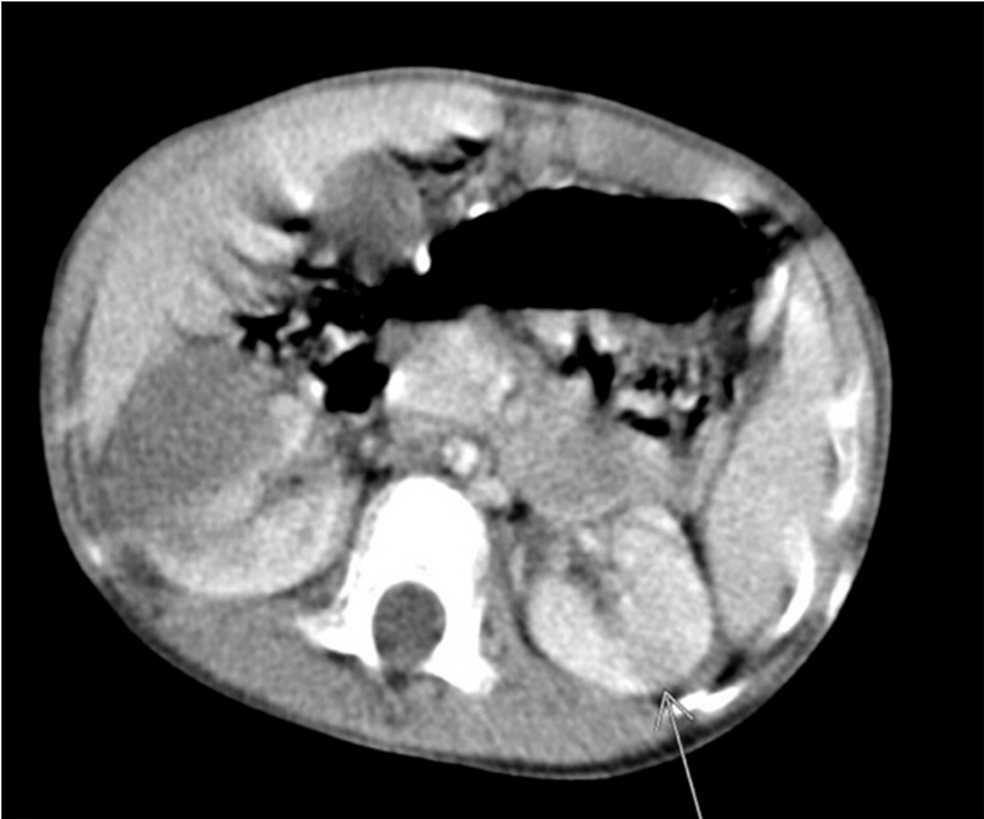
CT scan after six cycles of chemotherapy showed a marked reduction in the right renal tumor and minimal changes in the left tumor (arrow indicates mass in the left kidney). CT: computed tomography
